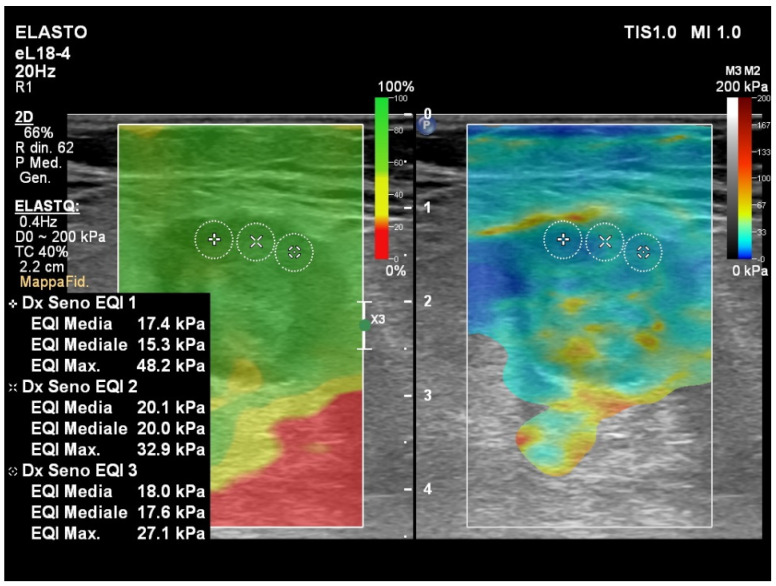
Intestinal ultrasound image showing a thickened terminal ileum with a prevalently hypo-echoic pattern at ultrasound shear-wave elastography (2D SWE) imaging.
